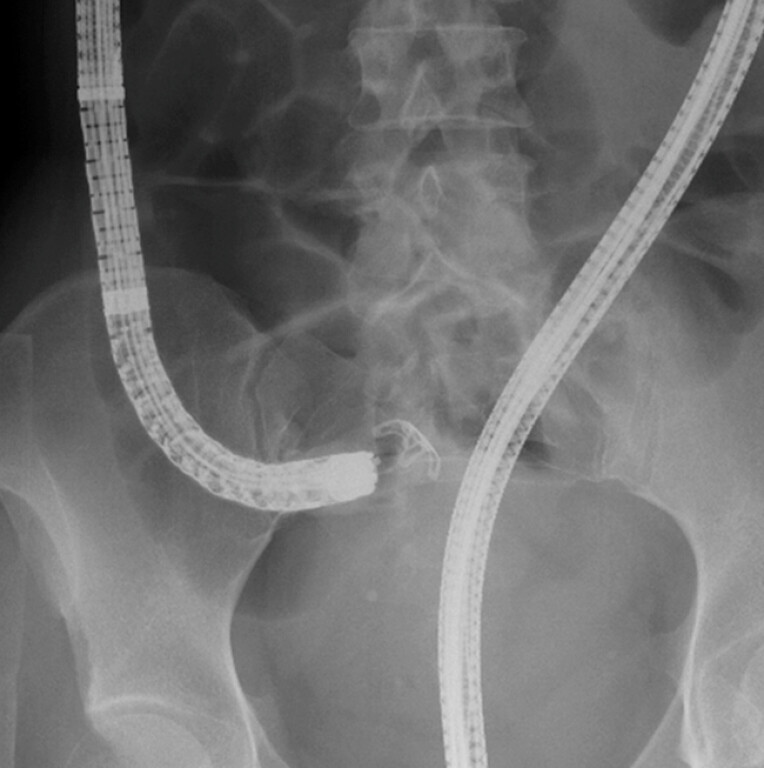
Radiographic image showing the deployment of the over-the-scope clip.
